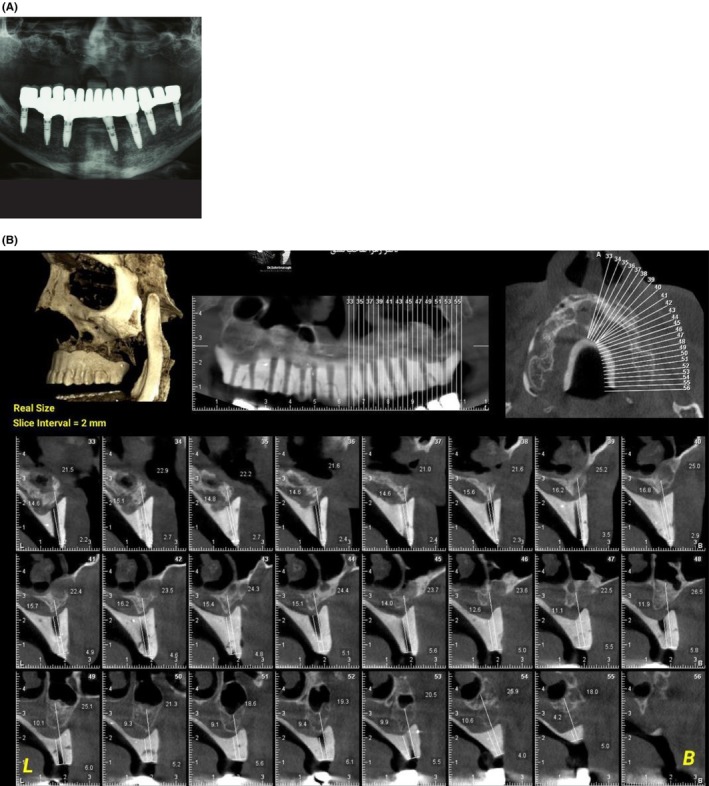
A: Panoramic radiography was taken 1 year after the initial implants failure. B: CBCT of maxilla with radiographic stent.
